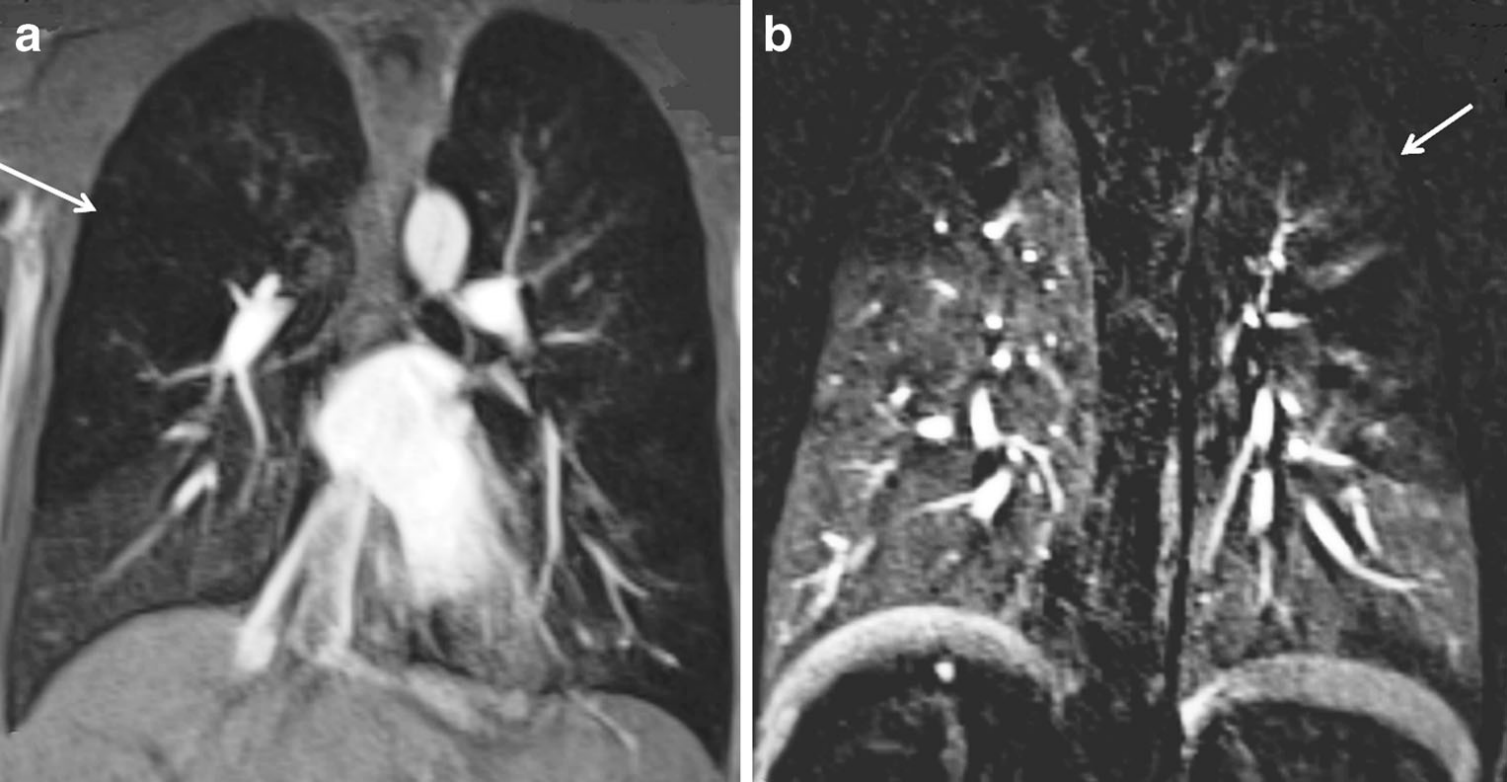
Contrast-enhanced MRI perfusion study in a 16-year-old boy with cystic fibrosis. Non-subtracted (a) and subtracted (b) coronal views. Note multiple areas of mosaic pattern likely representing areas of hypoperfusion (*arrows*)
